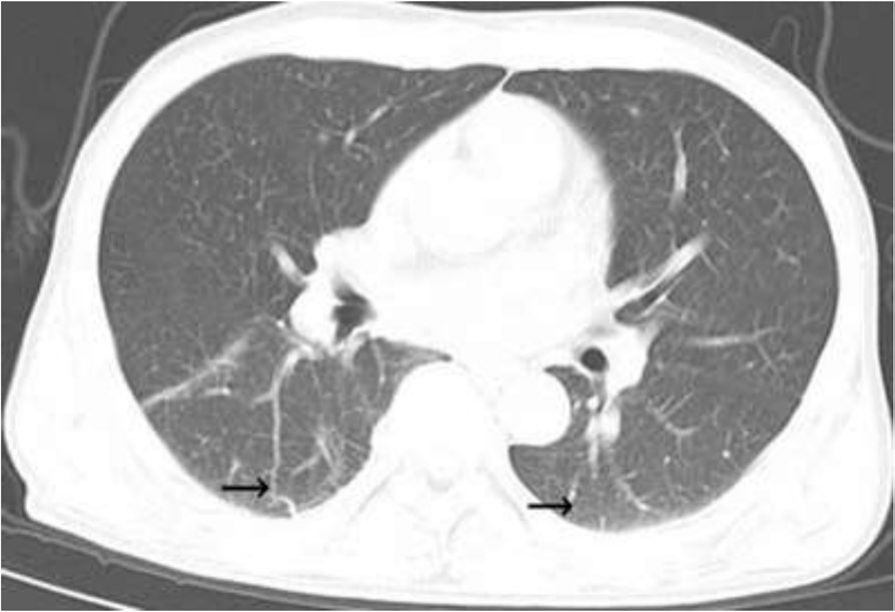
Lung CT scan. Lung CT scan imaging at February 2023. → signs of pulmonary diffuse inflammation
